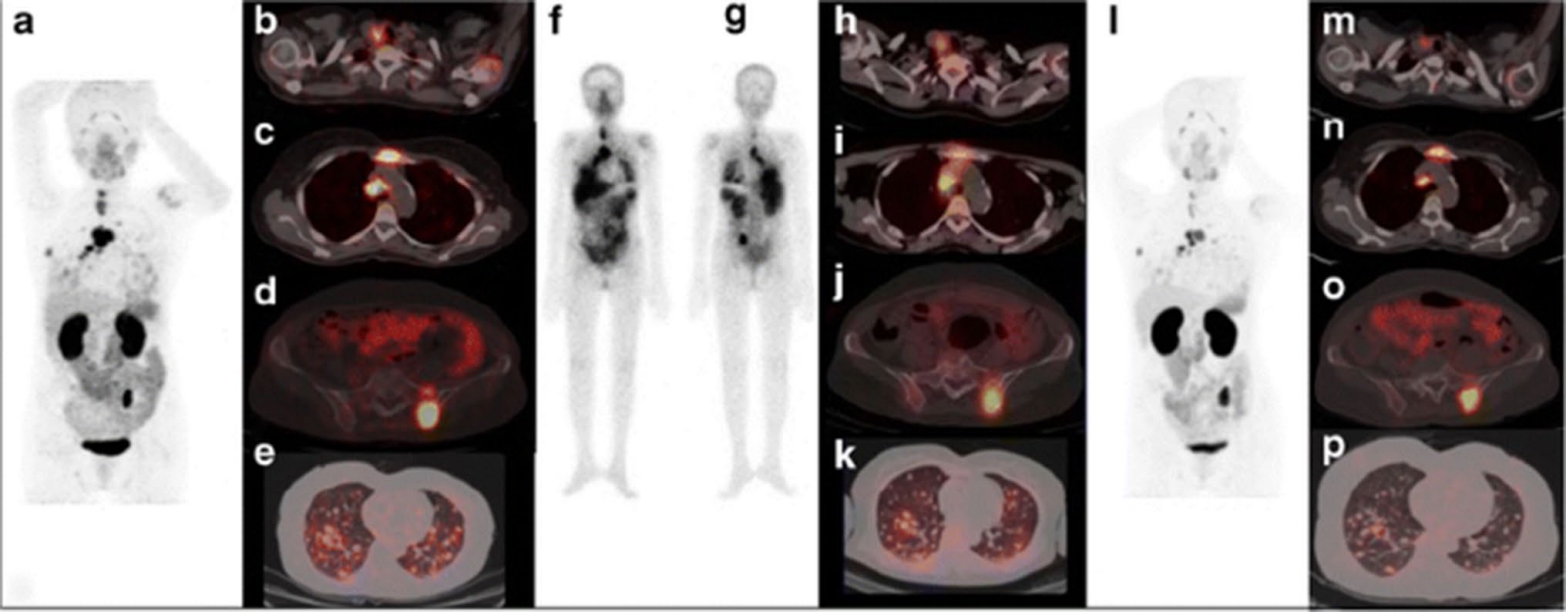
The maximum intensity projection (MIP) image of [^68^Ga]Ga-DOTA-E[c(RGDfK)]_2_ PET/CT for pretreatment assessment (a) and transaxial fused PET/CT images showed increased tracer uptake in the thyroid remnant [maximum standardized uptake value (SUVmax) = 4.7] with cervical lymph nodes (b), mediastinal lymph node (c; SUVmax = 8.4), lytic skeletal lesions with soft tissue component in the sternum (c; SUVmax = 7.8) and left iliac bone (d; SUVmax = 8.4) and multiple lung nodules (e). The patient received 5.5 GBq of [^177^Lu]Lu-DOTA-E[c(RGDfK)]_2_ with post-therapy whole-body images in anterior (f) and posterior (g) views revealing the overall distribution of [^177^Lu]Lu-DOTA-E[c(RGDfK)]_2_
